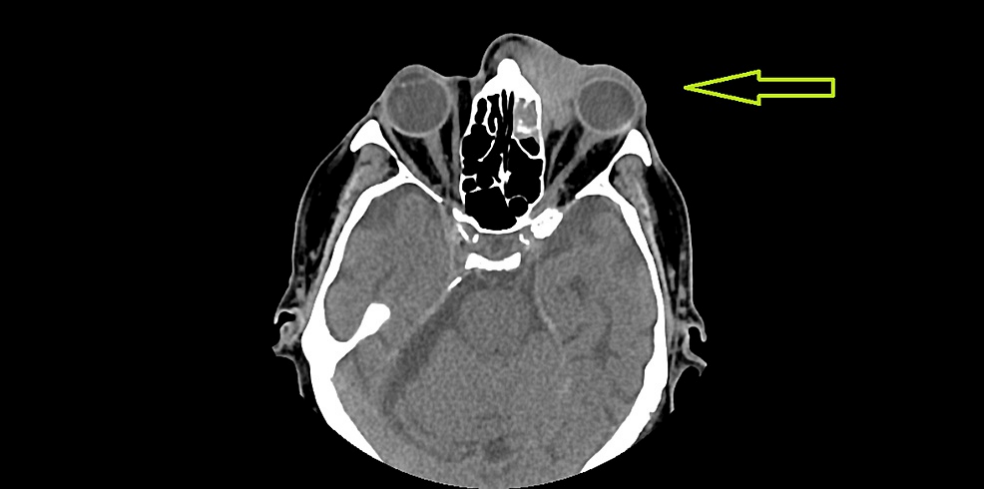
CT scan showing orbital mass affecting medial rectus muscle.
